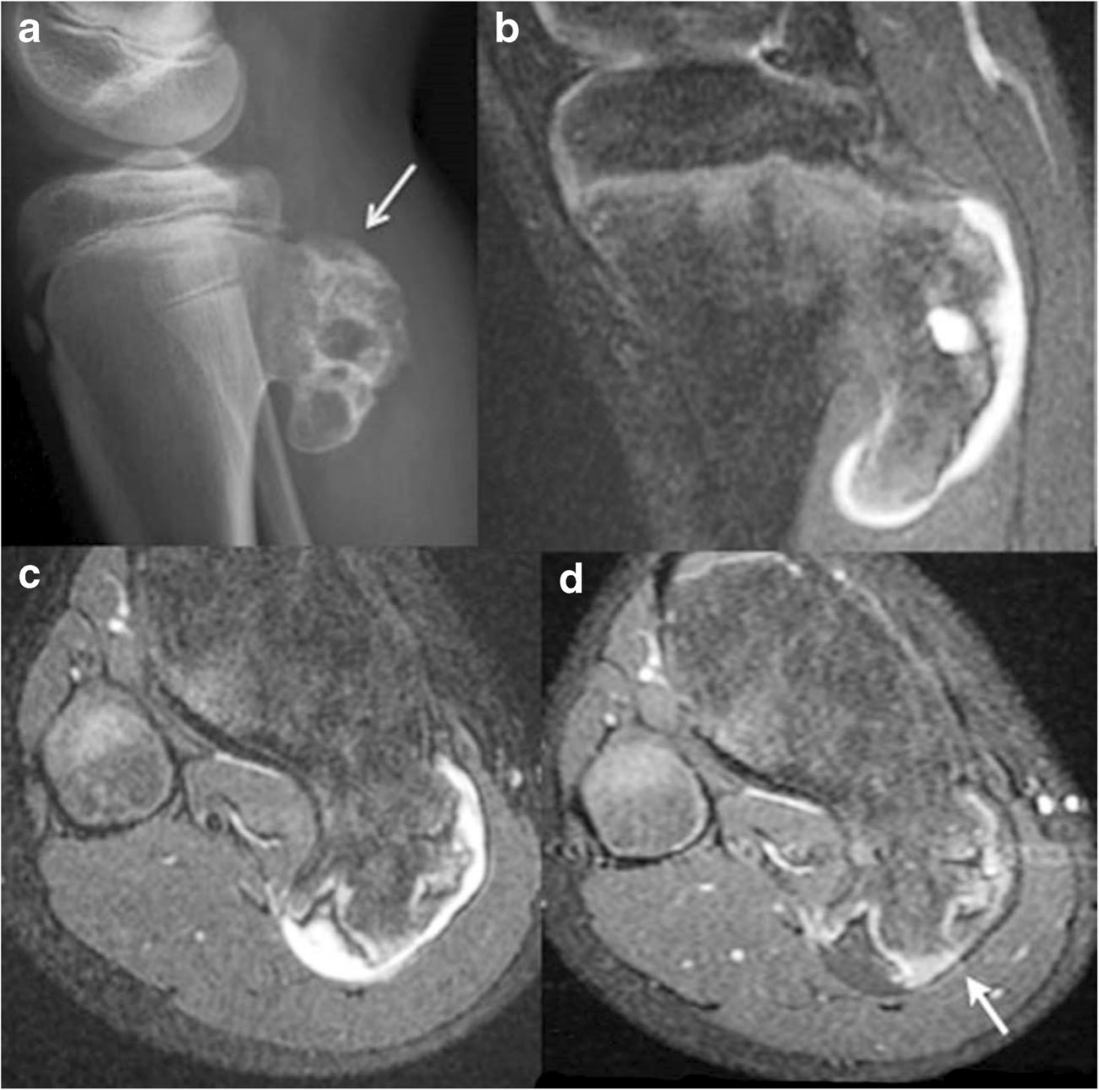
Benign solitary osteochondroma of the tibia in a 15-year-old boy. Lateral  radiograph of the knee a shows a bony protrusion arising off the posterior aspect of the proximal tibial metaphysis; note the continuity with the medullary cavity of the underlying tibia (*arrow*). Sagittal b and axial c T2 MR images show a markedly hyperintense, thin cartilage cap surrounding this bony exostosis; an axial T1 post contrast MR image d reveals a thin, peripherally enhancing cartilage cap (*arrow*)Subperiosteal osteoid osteomaOsteoid osteomas are benign bone-forming neoplasms, which account for approximately 13 % of benign bone tumours [10]. These occur mostly through the second and third decades of life. Broadly, these are classified based on location into medullary, intracortical and subperiosteal lesions. It is believed that many osteoid osteomas are, in fact, subperiosteal to begin with and later migrate into intracortical, endosteal or even medullary locations, secondary to continued bone remodeling, subperiosteal deposition and endosteal erosion [11].Radiographic findings include a circumscribed ovoid radiolucency or nidus measuring less than 1.5 cm, and surrounded by variable degrees of reactive bone formation and periosteal reaction (Fig. 4).
